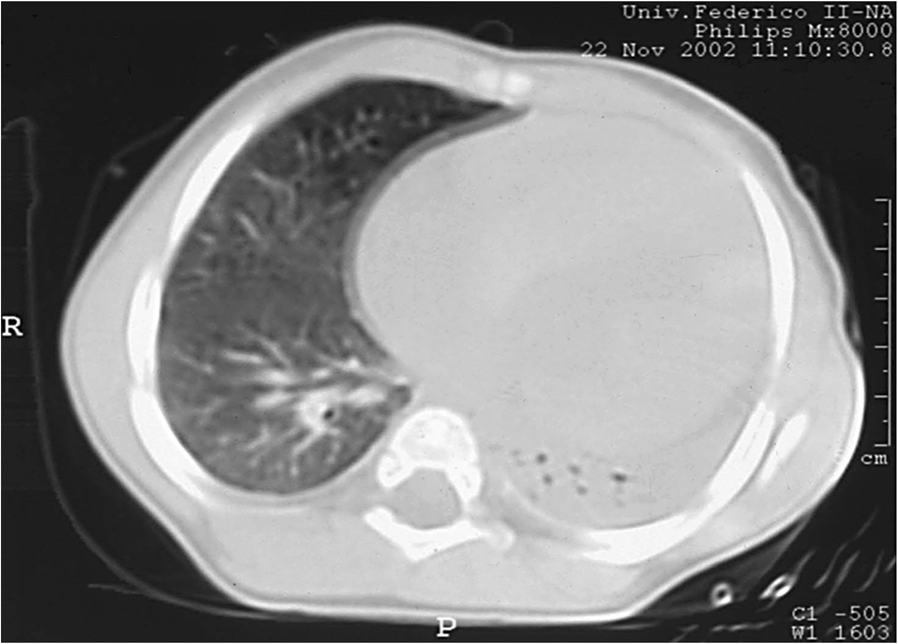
High-resolution computed tomography of the lung, showing massive atelectasis of the left lung with signs of intraparenchymal air trapping.
